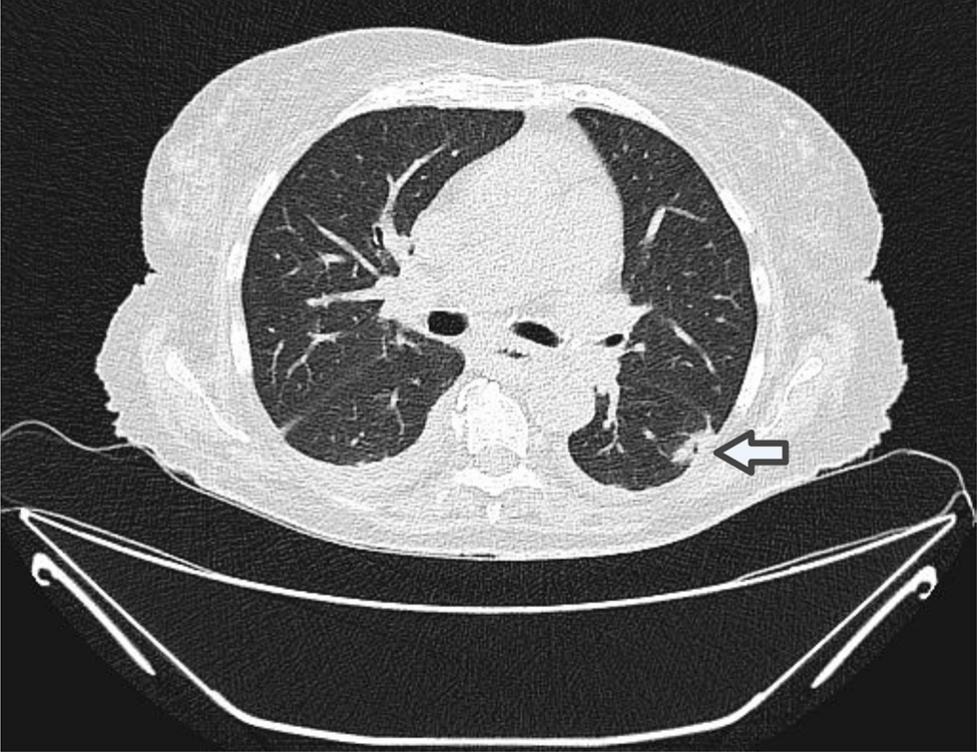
Chest computed tomography scan from an 82-year-old female patient with acute myeloid leukemia in study of *Bjerkandera* spp. pulmonary infection in immunocompromised hosts, Germany. Image depicts nodular infiltrates and a cavitory lesion (arrow) in the left lower lobe suggestive of fungal pneumonia. We cultured and identified a mold from bronchoalveolar lavage fluid as *Bjerkandera* spp. by internal transcribed spacer sequencing.
